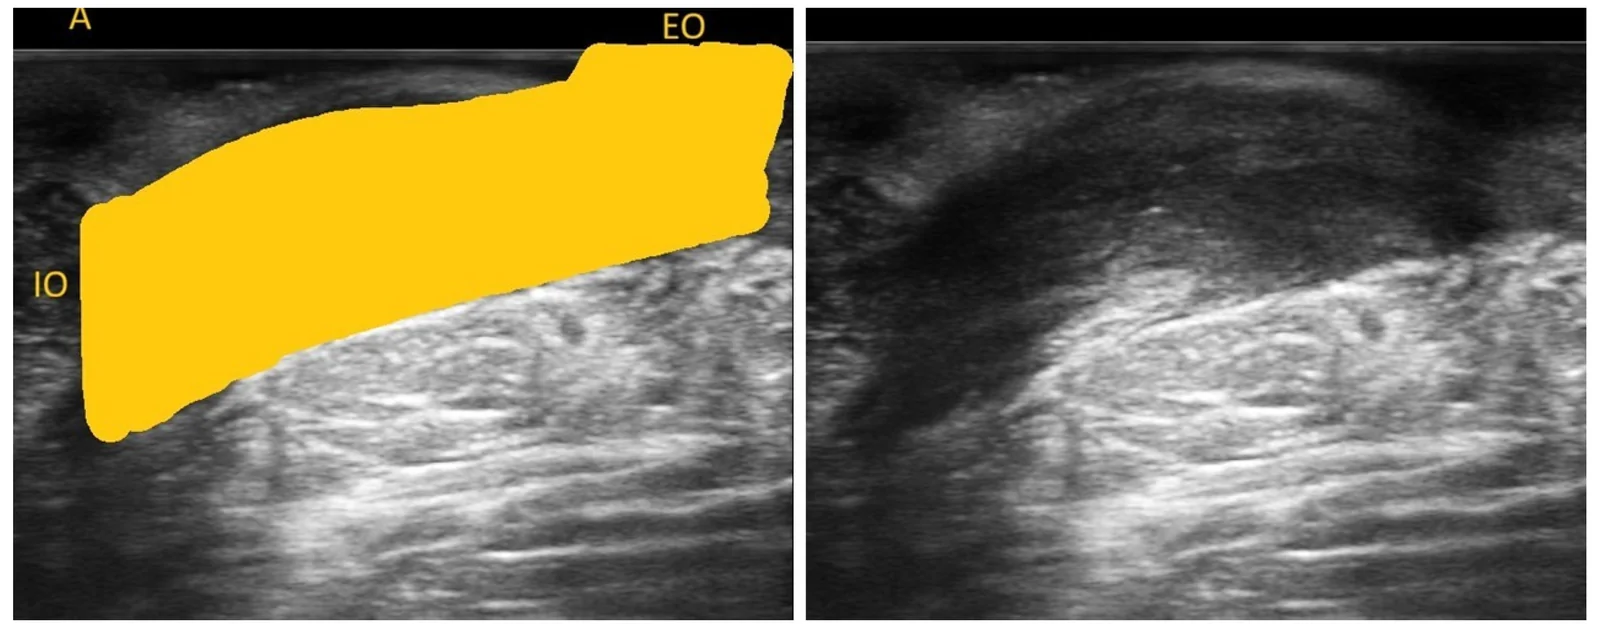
An ultrasound image obtained with a superficial linear probe (7.5 MHz) shows a simple low-type perianal fistula with a fistulous tract measuring 5 cm in length, with an obvious external opening (EO) and a single internal opening (IO) into the anal canal (AC) located 14 mm away from the anal verge (A). No fistulous branching or abscess. The left image shows the tract of the fistula (thick yellow line), EO, and IO. The right image is the corresponding unannotated scan.
